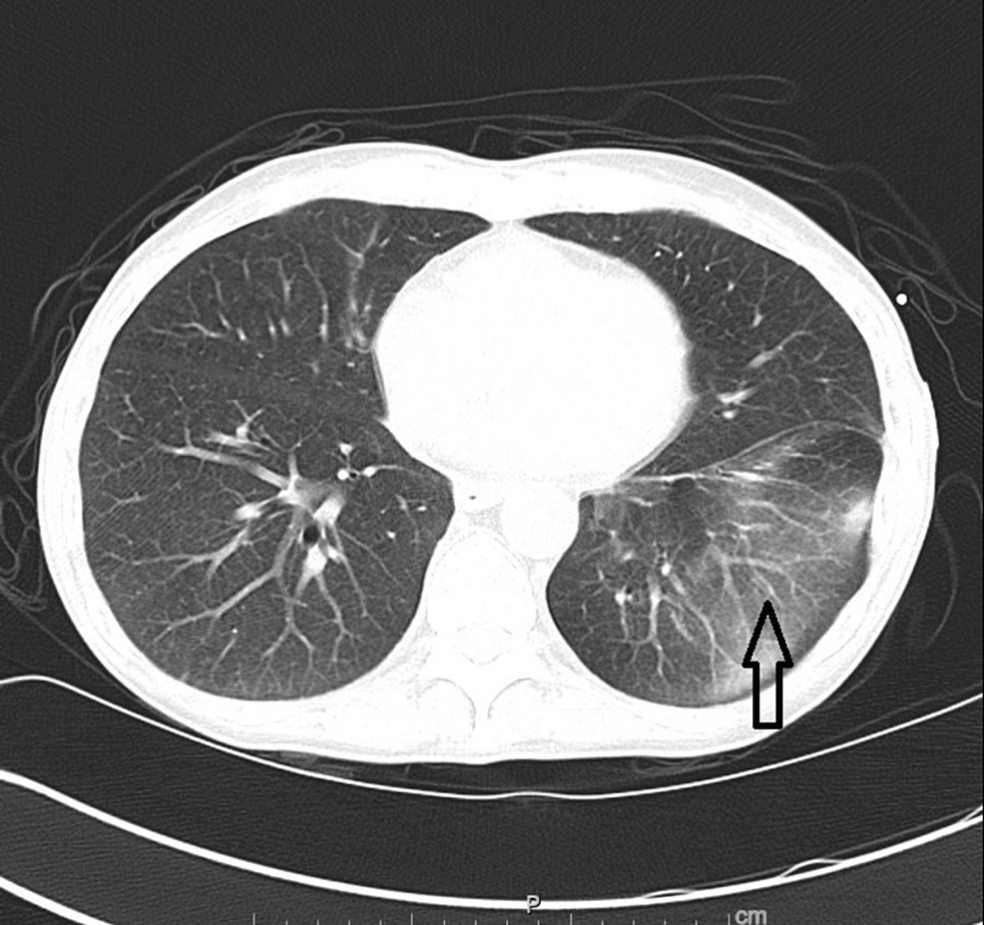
CT scan of chest. Chest CT scan showing faint ground glass opacities throughout the lung (arrow).
